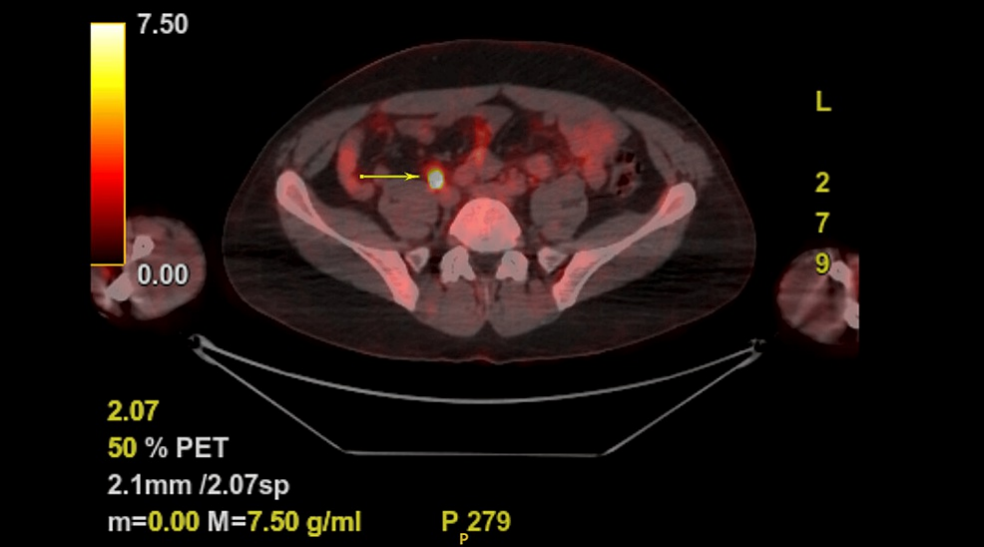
Fused axial PET/CT corresponding to a lesion in the rectum (arrow), which proved to be the site of the 2-3 cm rectal mass.
